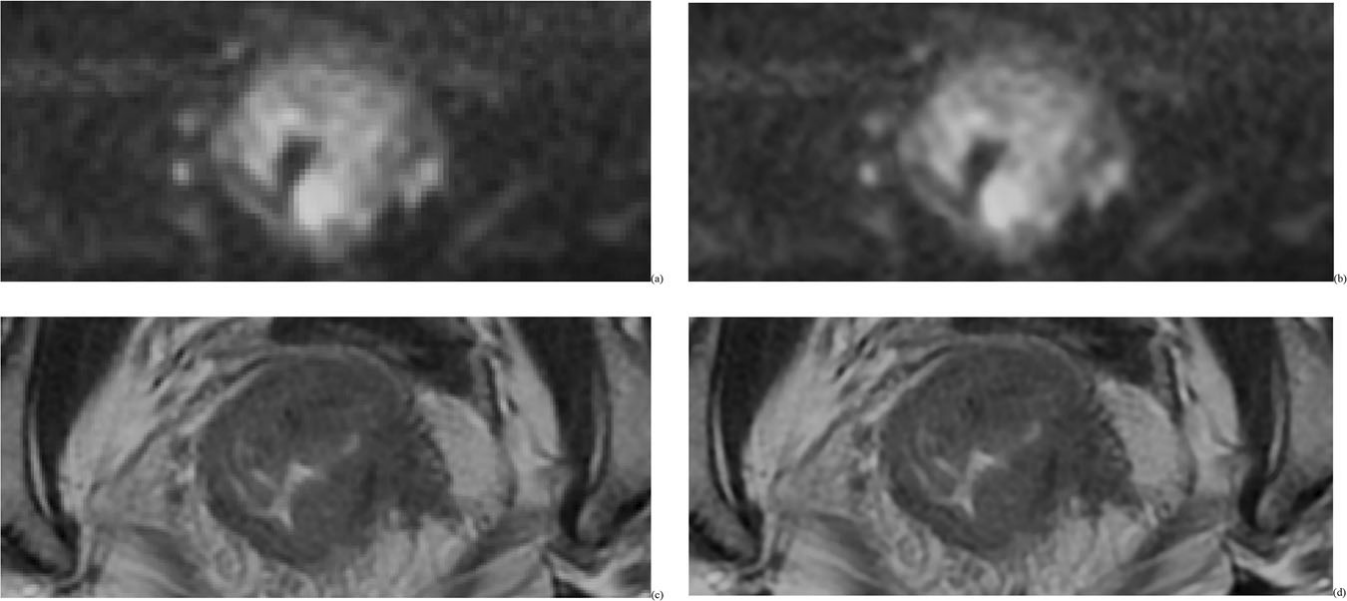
It shows the SR and ORI images: a ORI-DWI image; b the SR-DWI image; c the ORI-T2WI image; d the SR-T2WI image
